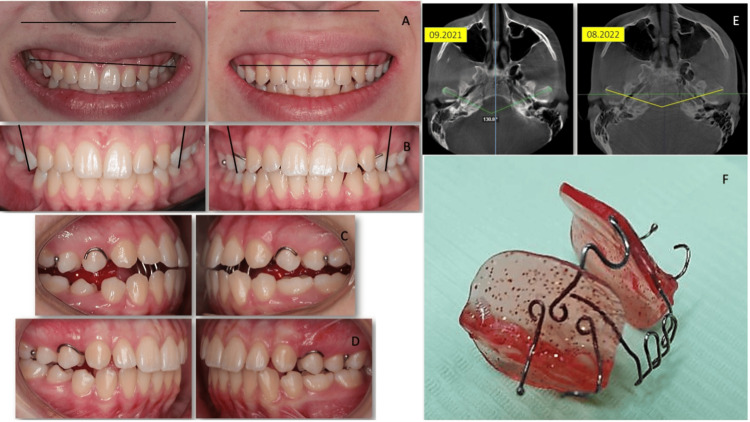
Jaw functional orthopedics treatment. Left panel: Occlusion of the patient before (A, B) and after Jaw Functional Orthopedics treatment (C, D). Right panel: Upper: CBCT images of the temporomandibular joint before and after treatment (E). Lower: Jaw Functional Orthopedics appliance (F). CBCT: cone beam computed tomography
